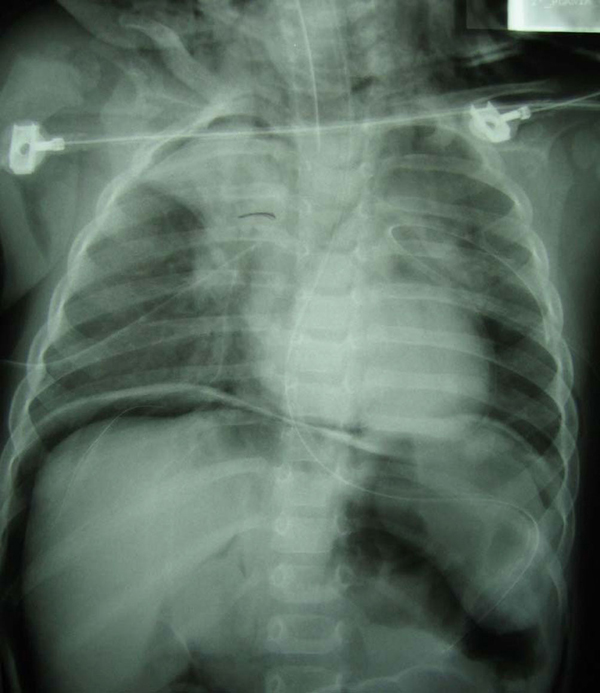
Thorax-abdomen radiography showing pneumopericardium, pneumoperitoneum, subcutaneous emphysema and right pneumothorax, with right upper lobe atelectasis. Chest tubes in both hemithoraces.
